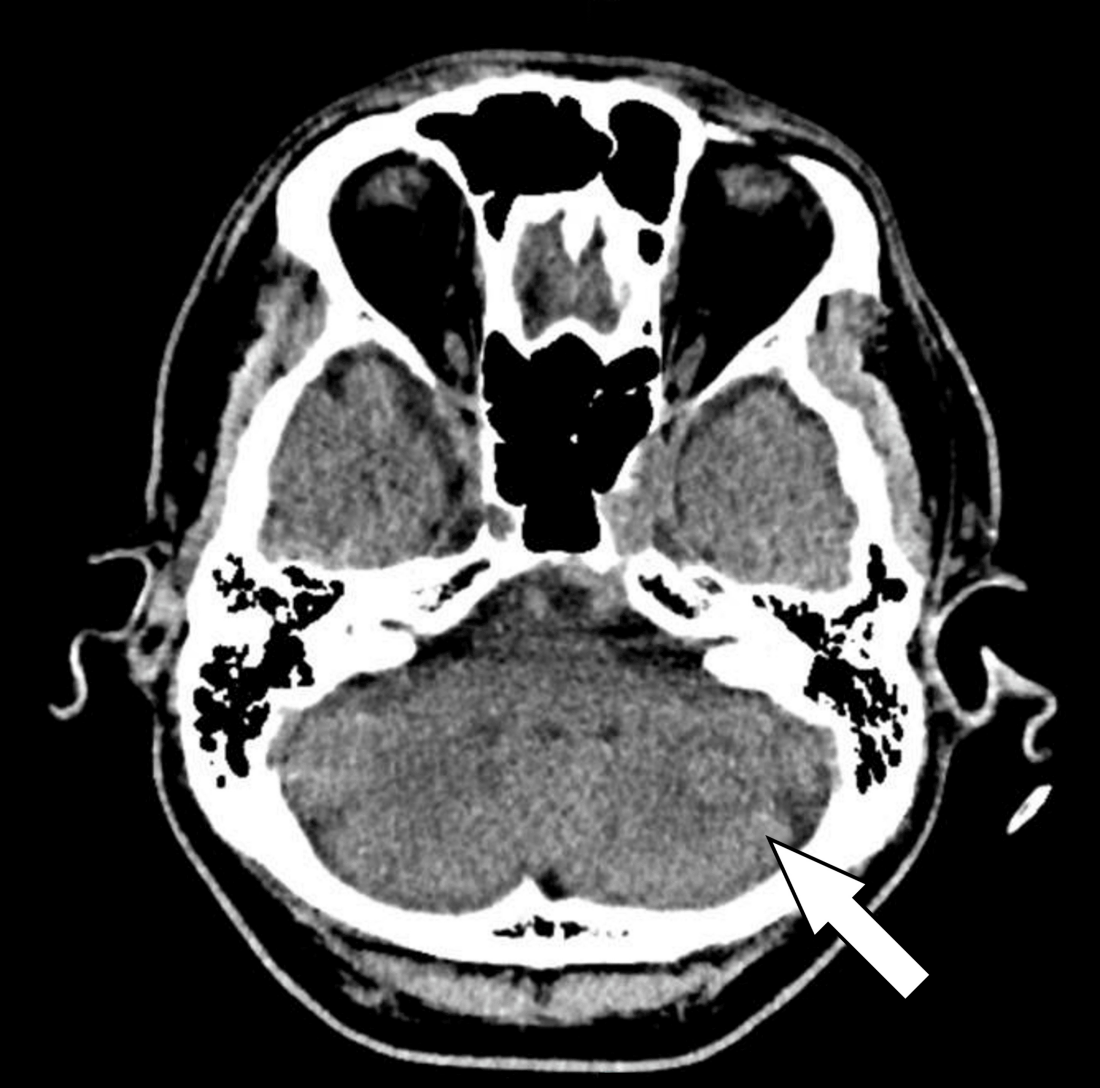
Axial view showing a left cerebellar lesion smaller in size compared to that in previous MRI brain
